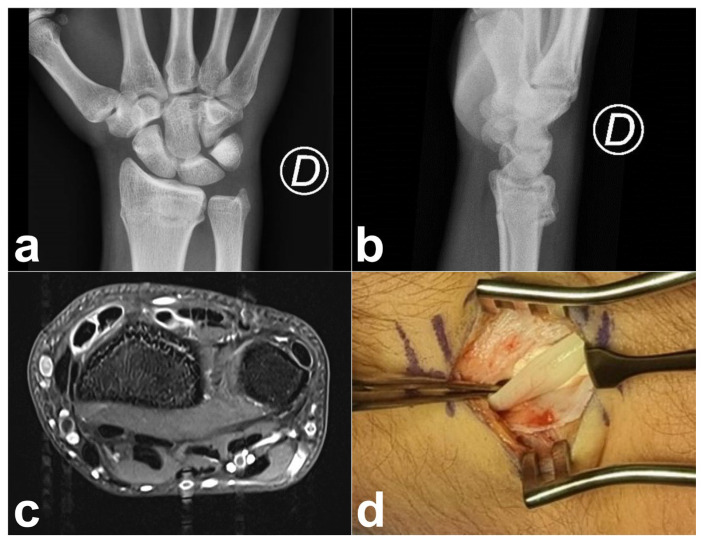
Case 5. (a,b) Preoperative radiograph showing a distal radial fracture with minimal dorsal tilt. (c) DIT: Tenosynovitis of the EPL, ECRB, and ECRL tendons of the right wrist on MRI (axial T1-weighted turbo spin-echo with fat saturation and Dixon-based reconstruction). (d) Perioperative photograph after teno-synovectomy and subcutaneous transposition of the EPL tendon.
